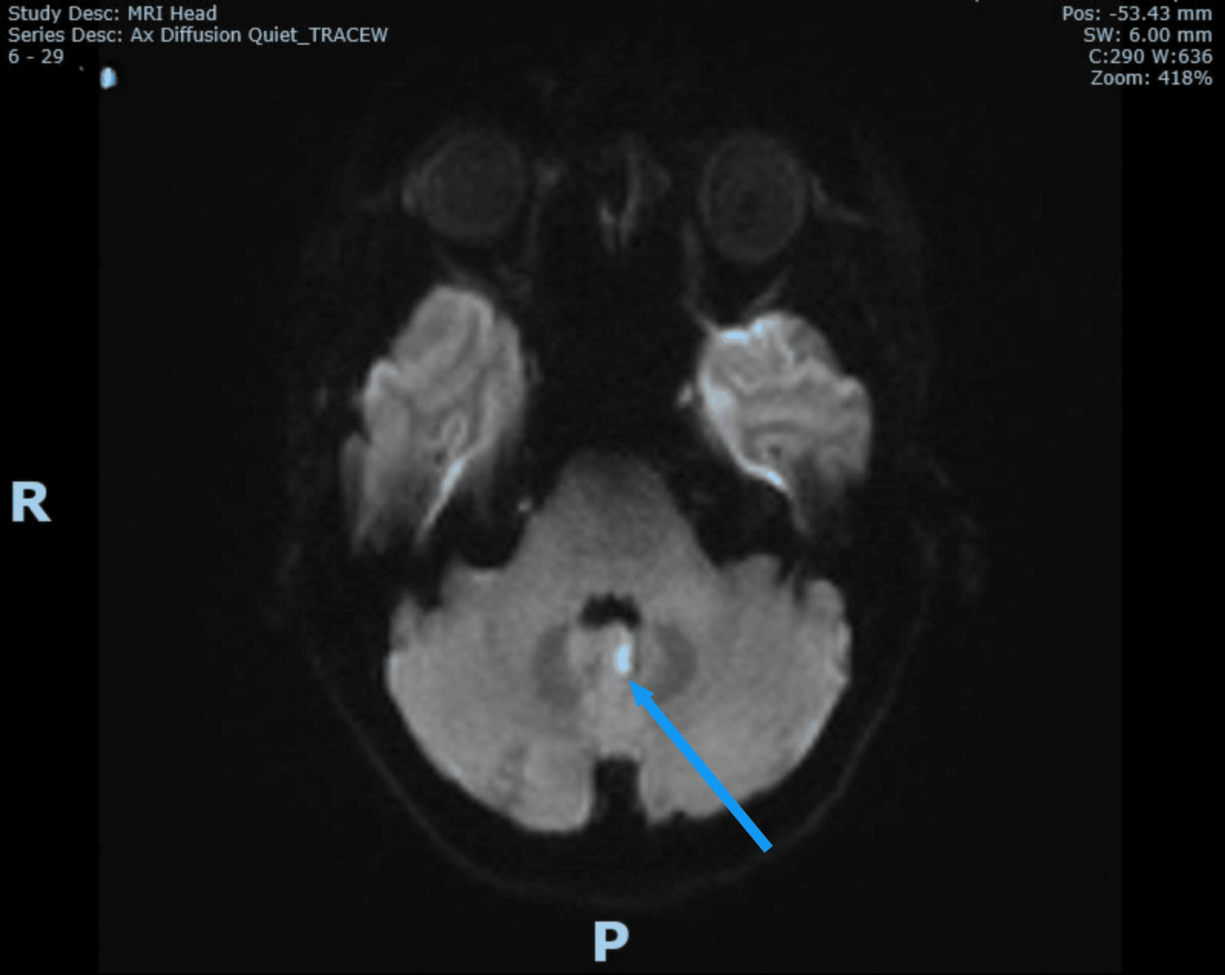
MRI brain DWI showing infarct at left cerebellar vermix (blue arrow) MRI: magnetic resonance imaging DWI: diffusion-weighted imaging
